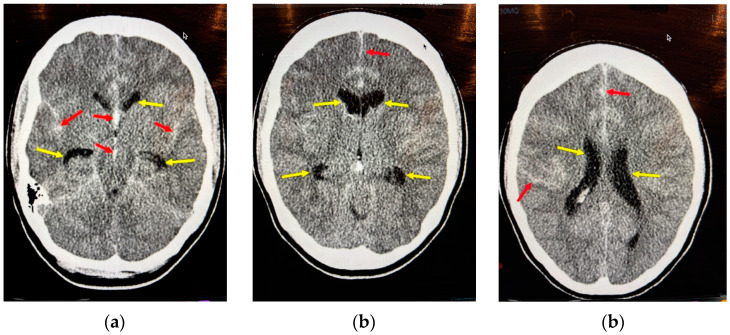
An example of acute hydrocephalus following aSAH secondary to a ruptured ACommA in a clinical aSAH grade 3 28-year-old woman. The red arrows point to subarachnoid blood in the cisterns, sulci, and interhemispheric fissure, and the yellow arrows point to prominent ventricles. This degree of hydrocephalus, while not florid, was clear with visible temporal horns (normally not visible in young adults) (a), and “rounding” of the frontal horns of the lateral ventricles (b). Combined with the flattening of the cerebral sulci (c), this CT scan is indicative of dangerously raised intracranial pressure, especially in a young patient requiring early EVD insertion.
